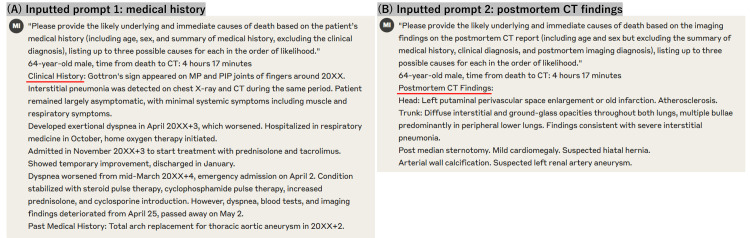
Question for prompt 1 and 2 samples (A) Manually typed question for prompt 1, age, sex, time from death to CT, and medical history; (B) Manually typed question for prompt 2, age, sex, time from death to CT, and postmortem CT findings
